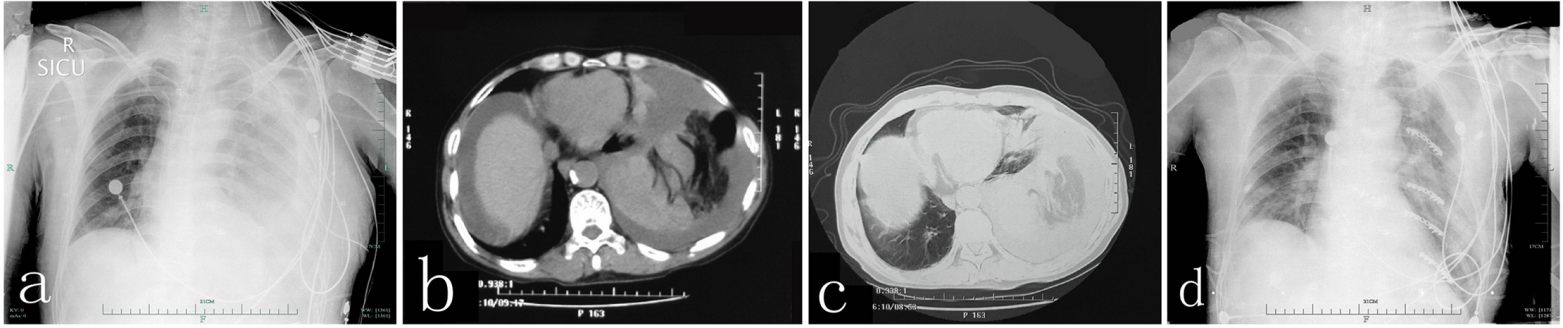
Pre-operative CR and CT (a, b and c) showed massive pleural effusion and the edge of the diaphragm was not clear; post-operative CR (d).
